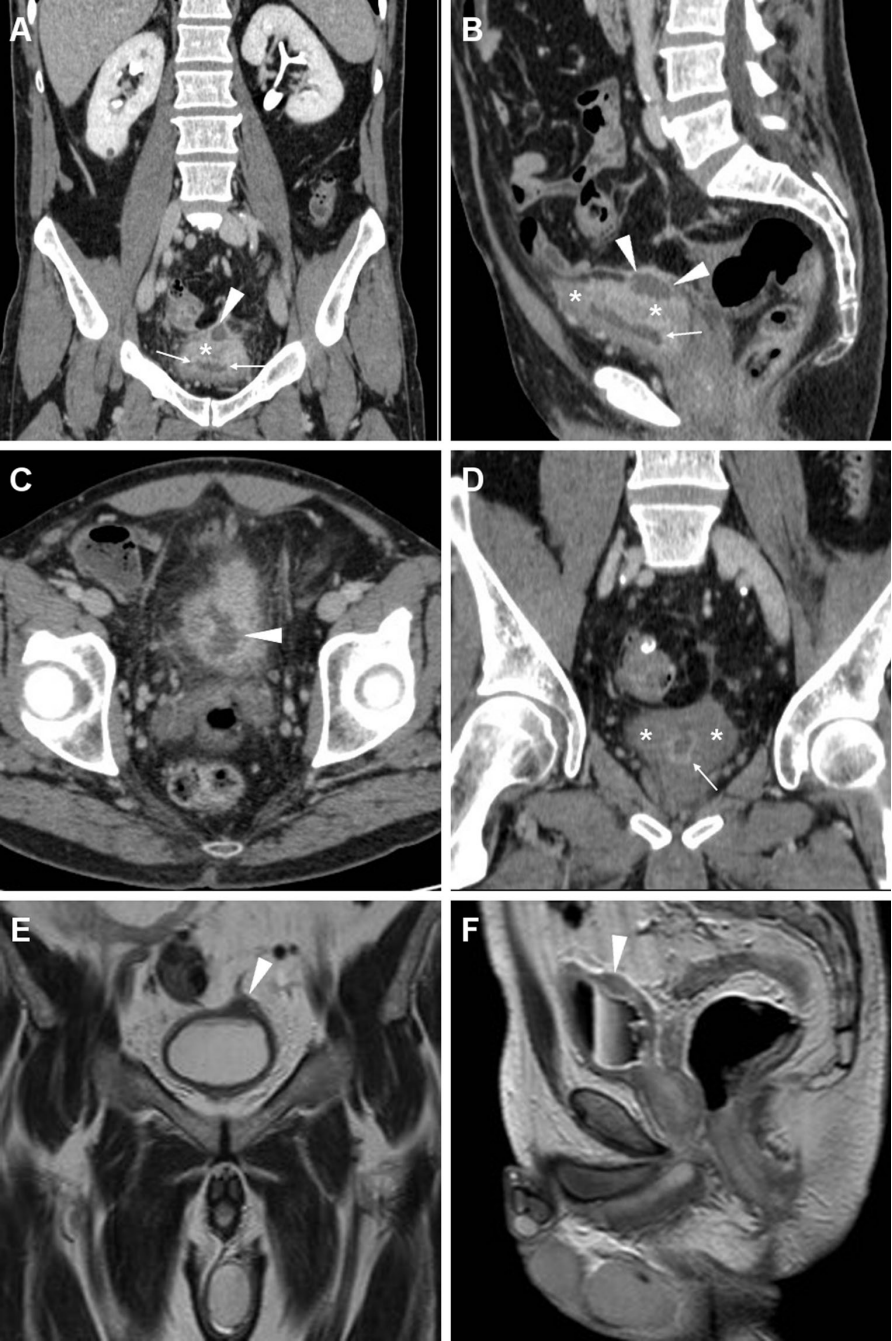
Mural bladder abscess in a 61-year-old man with recurrent UTIs and clinical and sonographic suspicion of bladder carcinoma. Multiplanar CT images (a–d) depicted a poorly distensible bladder with mural thickening (*), urothelial hyperenhancement (*thin arrows*) consistent with active UTI, and a fluid-like collection (*arrowheads*) with irregular peripheral enhancement along the posterolateral aspect. Urine cytology and cystoscopy excluded the presence of tumour. Six weeks later, repeated CT (d) after antibiotic treatment showed resolved abscess, persistent mural thickening (*) and infectious urothelial enhancement (*thin arrow*). Further follow-up with MRI including T2- (e) and post-gadolinium T1-weighted (f) sequences confirmed abscess disappearance (*arrowheads*)
